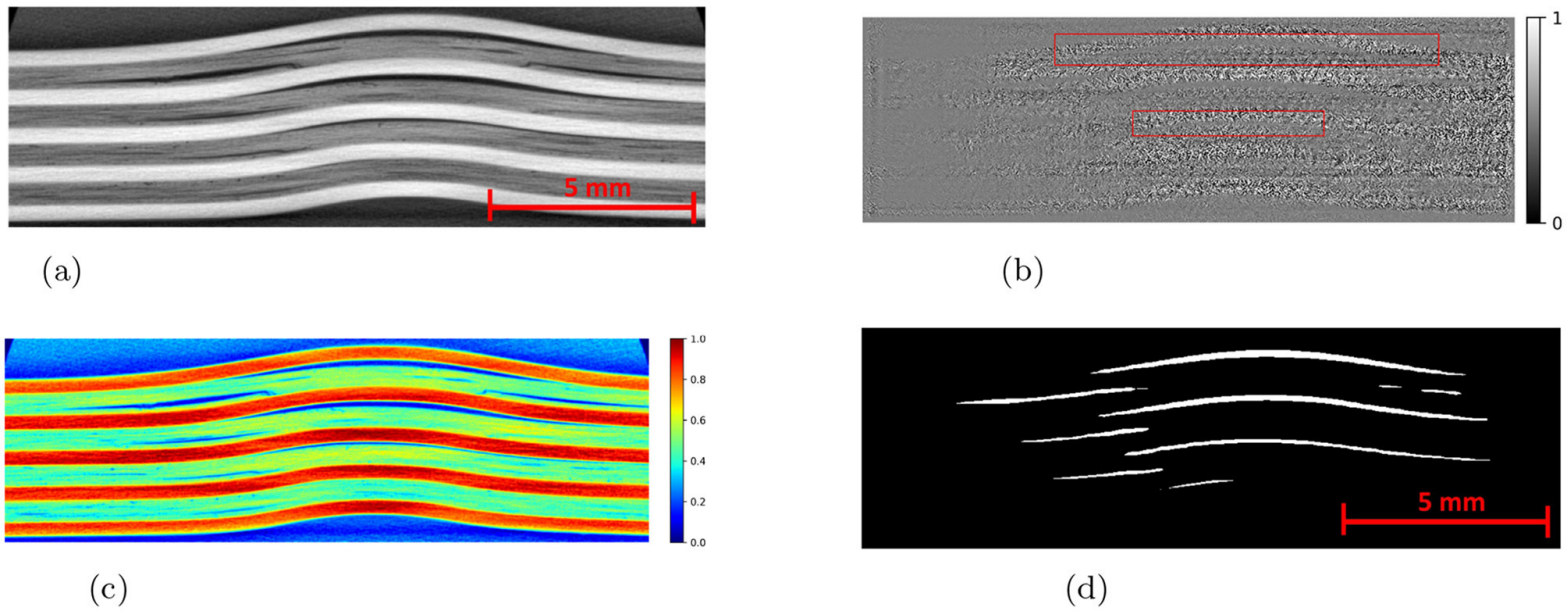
Visualization of IG results for impact energy of 10 Joules. (c) Shows the attribution map produced using the IG method with the modified loss function applied on the trained Mask R-CNN model, using the CT slice of the FML plate shown in (a). Example red boxes shown in attribution map (b) highlight attribution of damages. (a) CT slice with impact energy of 10 Joules. (b) Attribution map overlay. (c) Attribution map (modified loss). (d) Predicted mask.
